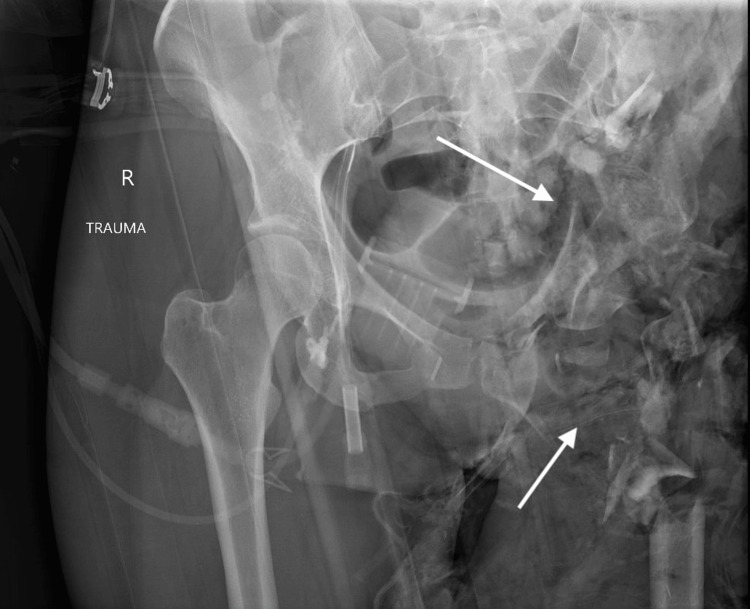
Pelvic X-ray The arrows indicate complete destruction of the hemipelvis and proximal femur.
